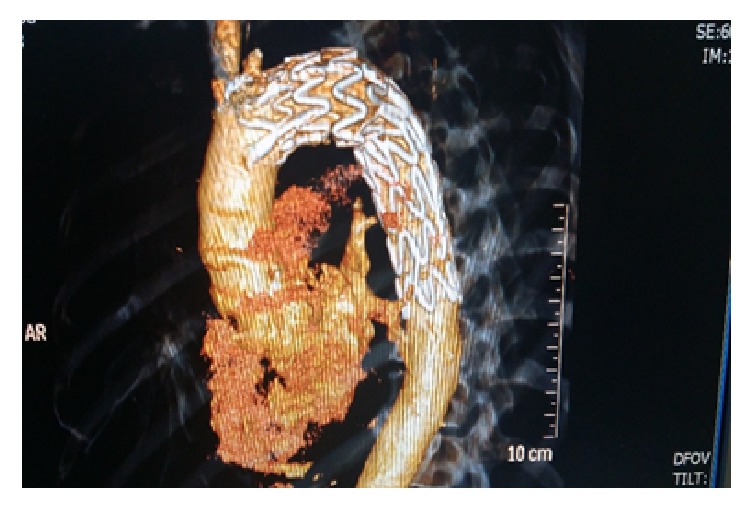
Three-dimensional CT angiography of thoracic aorta with proper placement of endograft and occlusion of left subclavian artery.
